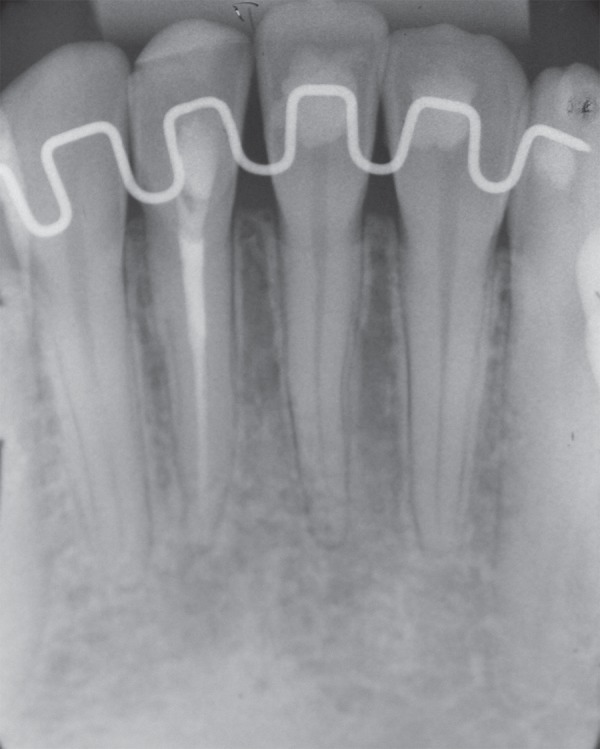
Control periapical radiograph of tooth #41 six months after endodontic
